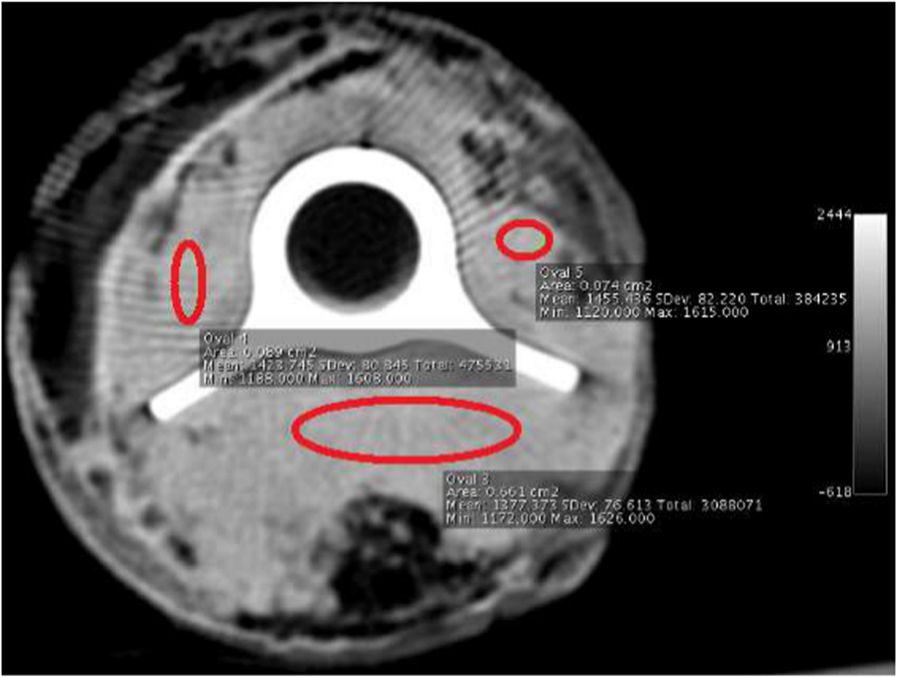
CT scan of a femoral head 3 mm beneath the cemented tibial tray. Three areas were selected, two beside the prosthesis and one posterior to the prosthesis.
